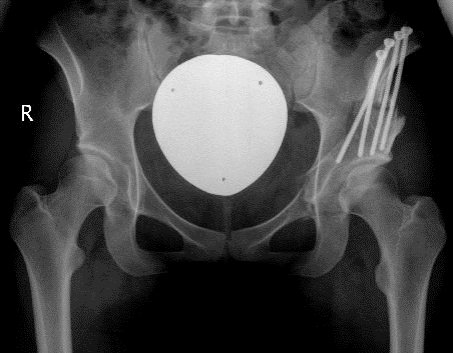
Postoperative radiographic evaluation after two-incision periacetabular osteotomy. Full correction and fixation of the acetabulum was achieved.
